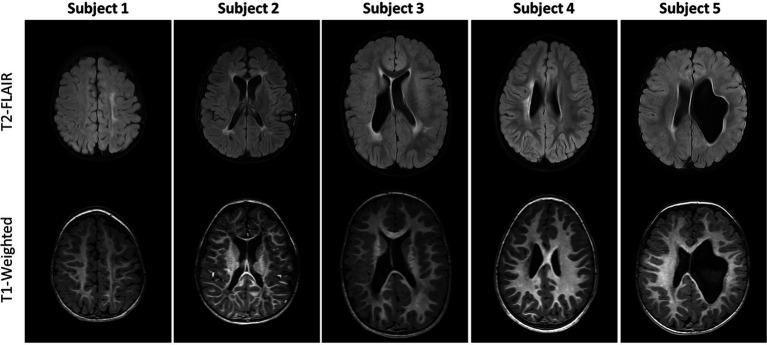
Axial T2-FLAIR (top row) and Axial unenhanced T1-weighted images (bottom row) for the five subjects studied. Different axial sections are shown so that each subject’s injuries are visible. MRI findings from these two sequences are as follows. Subject 1: Mild left white matter injury of centrum semiovale (CS), minimal left perirolandic volume loss and cortical/subcortical gliosis. Subject 2: Moderate bilateral periventricular leukomalacia (PVL) and white matter injury of the centrum semiovale, moderate volume loss and perirolandic cortical/subcortical gliosis; Subject 3: Moderate right, mild left PVL and white matter injury of the centrum semiovale, mild right volume loss; Subject 4: Moderate white matter injury of right centrum semiovale, mild volume loss and perirolandic cortical/subcortical gliosis; Subject 5: Moderate/Severe left volume loss, minimal bilateral PVL.
